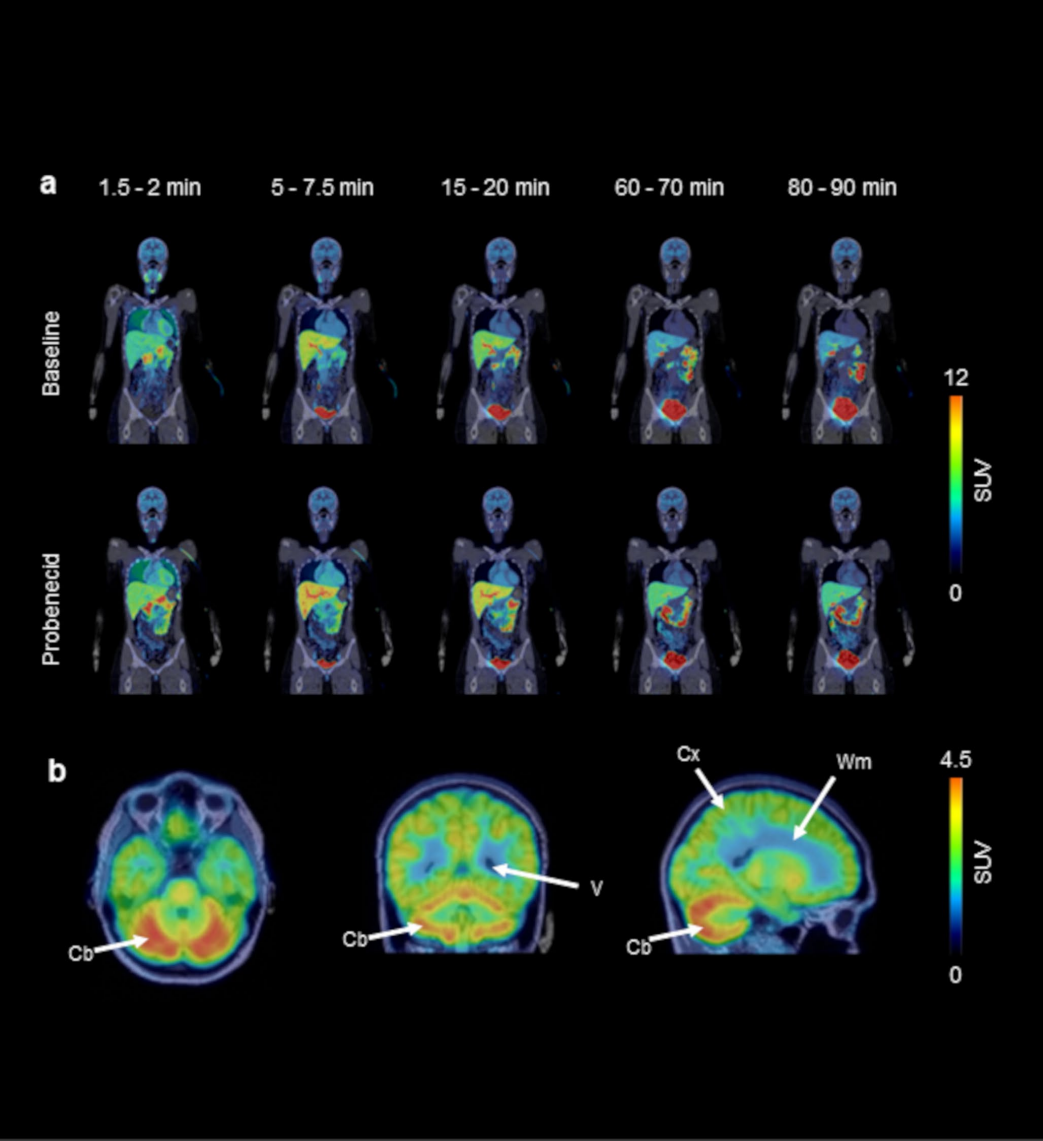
Coronal whole-body PET/CT images obtained at different time points after injection of [^11^C]BMP in one representative female subject for the baseline scan and the scan after probenecid administration (a) and MR-co-registered PET average images (0–90 min) of the brain in horizontal, coronal and sagittal planes of the same subject for the baseline scan (b). In b, anatomical structures are labelled with white arrows (Cb, cerebellum; Cx, cortex; Wm, white matter; V, left lateral ventricle)
